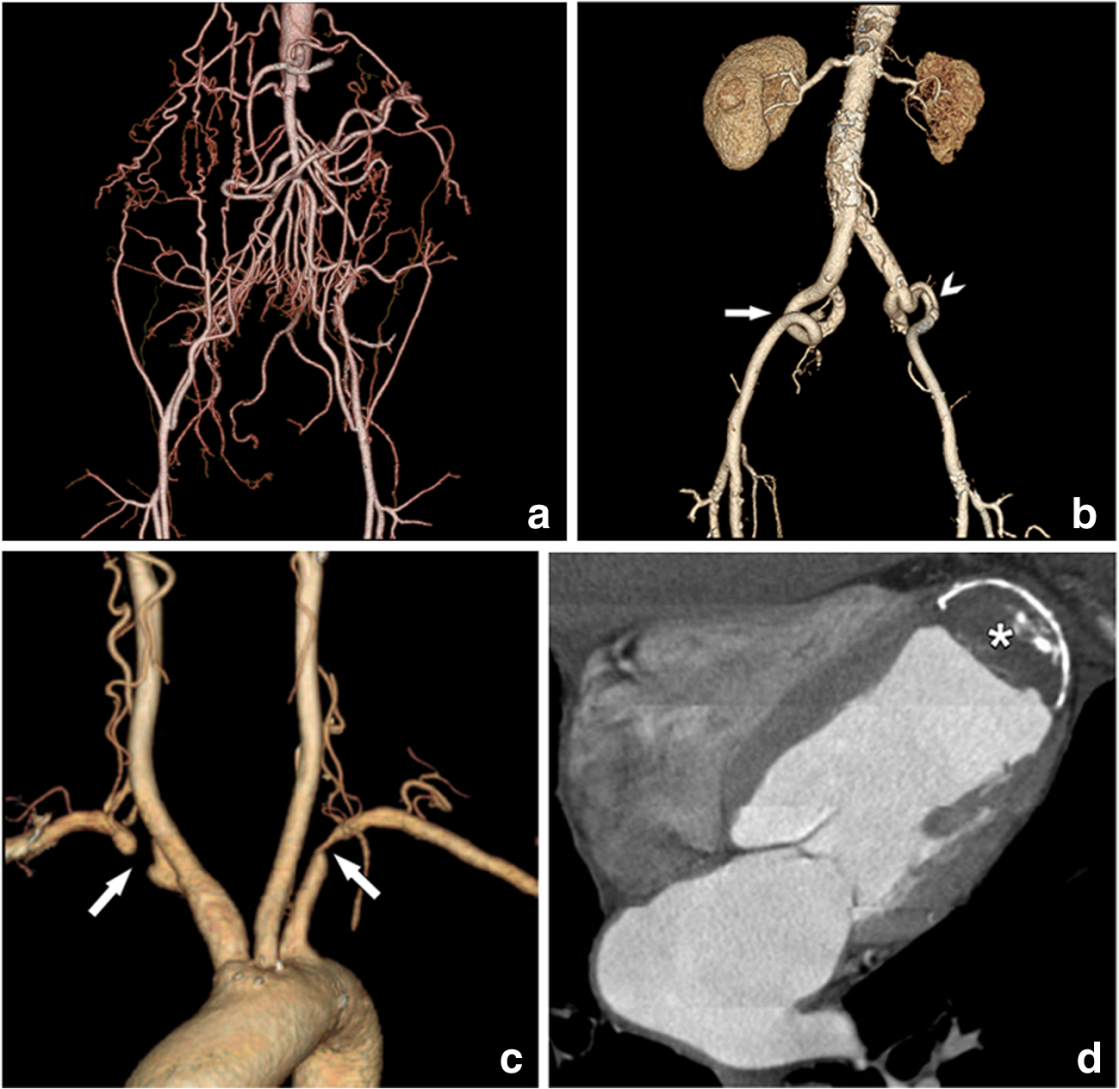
Compromised delivery paths. A safe endovascular trajectory is needed for safe transportation of the THV to the aortic root. CT is in his respect an essential tool in order to avoid vascular complications and guide to the intervention through the safest possible passage. Potential complications may arise due to luminal narrowing or even chronic iliac artery occlusion with extensive collaterals (a) and pronounced vascular tortuosity and kinking (arrows in b). In this last case, there is an additional short dissection in the left external iliac artery (arrowhead) due to a previously performed conventional coronary angiography. For these patients, the preferred transfemoral access approach is therefore not possible, and other options have to be considered. Nevertheless, other access paths may also pose significant challenges, like bilateral subclavian artery narrowing and occlusion (arrows in c), and the presence of post-infarct thrombus and wall calcification in the left ventricular apex (asterisk in d), making a transapical approach with a balloon-expandable valve impossible. Therefore, vascular access examination must include all anatomic possible entry points for a full assessment of the different options
